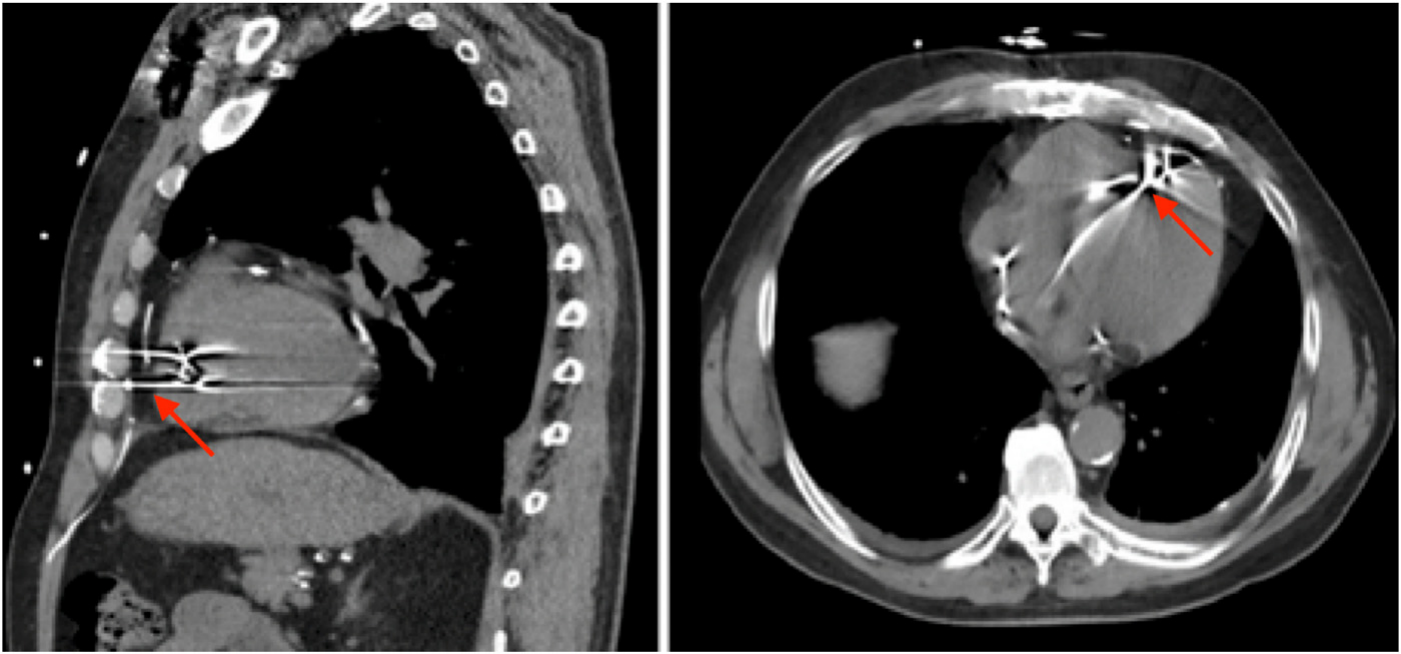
Chest Computed Tomography of Pacemaker Lead Perforation The images display a lead tip perforating the right ventricular myocardium and pericardium (red arrows).
